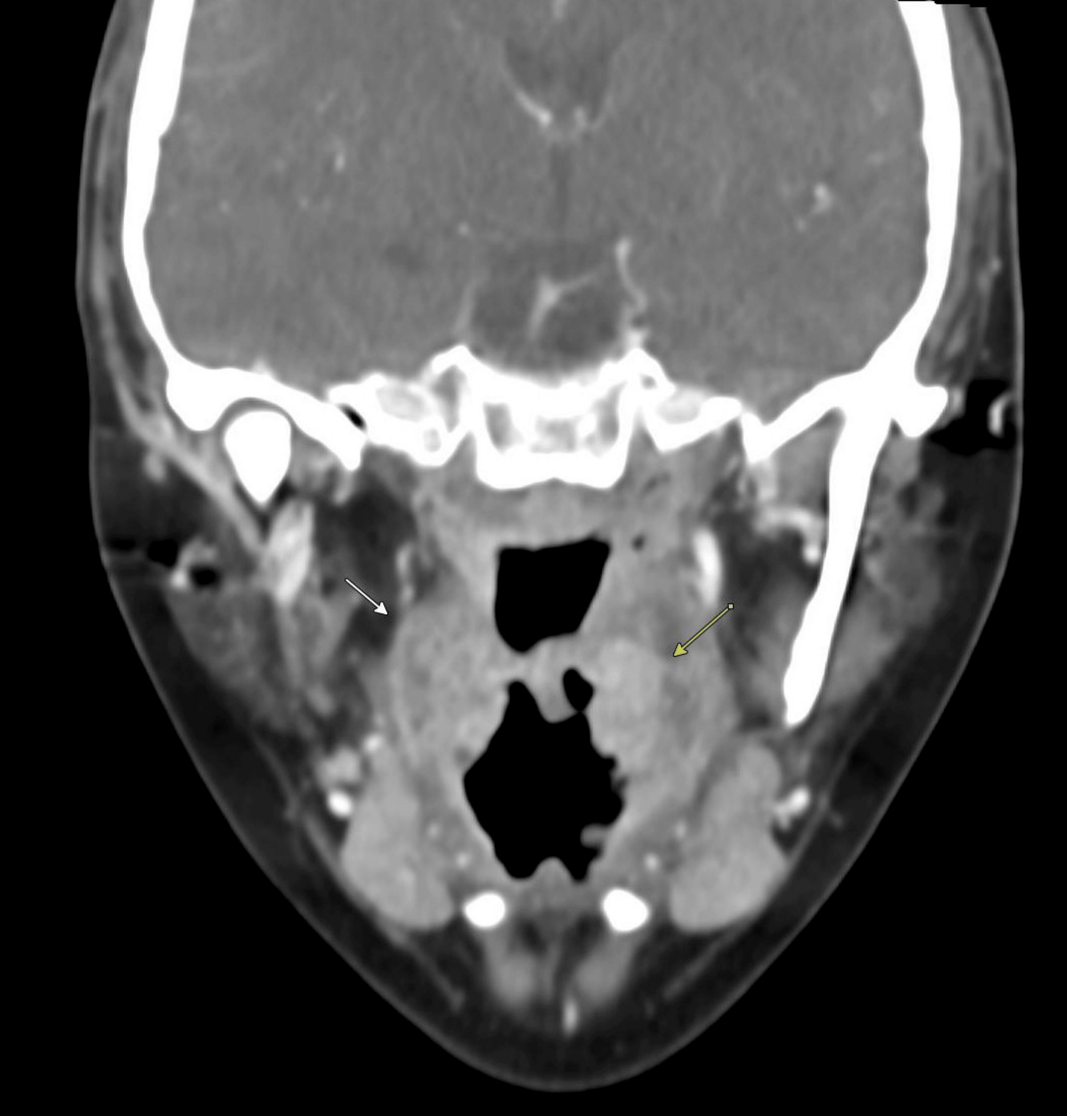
Coronal computed tomography image demonstrating bilateral palatine tonsillar enlargement consistent with acute tonsillitis (arrows)
